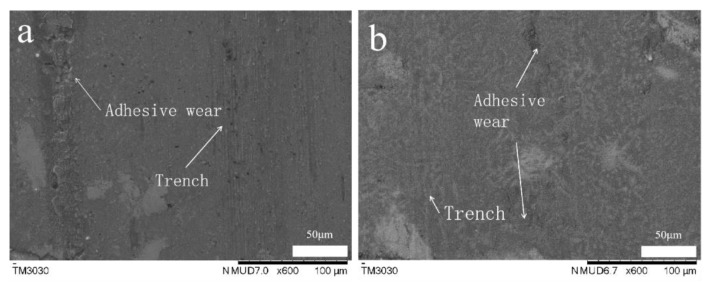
SEM photographs of abrasion marks on the fused cladding layer with different ultrasonic vibration frequencies applied: (a) 0 kHz and (b) 32 kHz.
